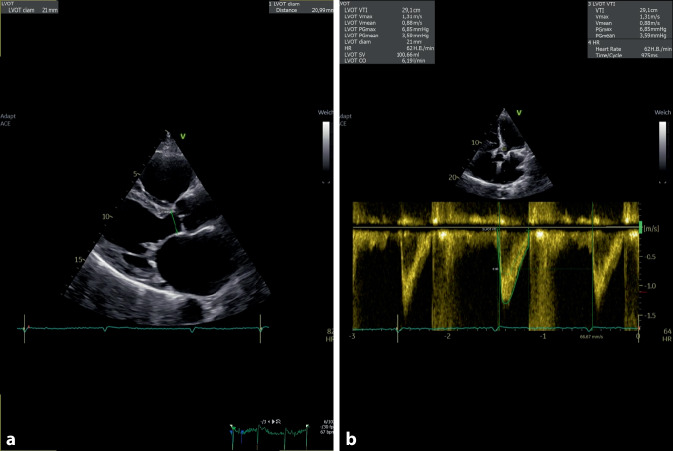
Bestimmung des Schlagvolumens bzw. Herzzeitvolumens (hier 6,2 l/min) durch Bestimmung des Durchmessers und des „velocity-time integral“ im linksventrikulären Ausflusstrakt. a Messung des LVOT Diameters in der parasternalen langen Achse, b Messung des VTI mittels PW Doppler
